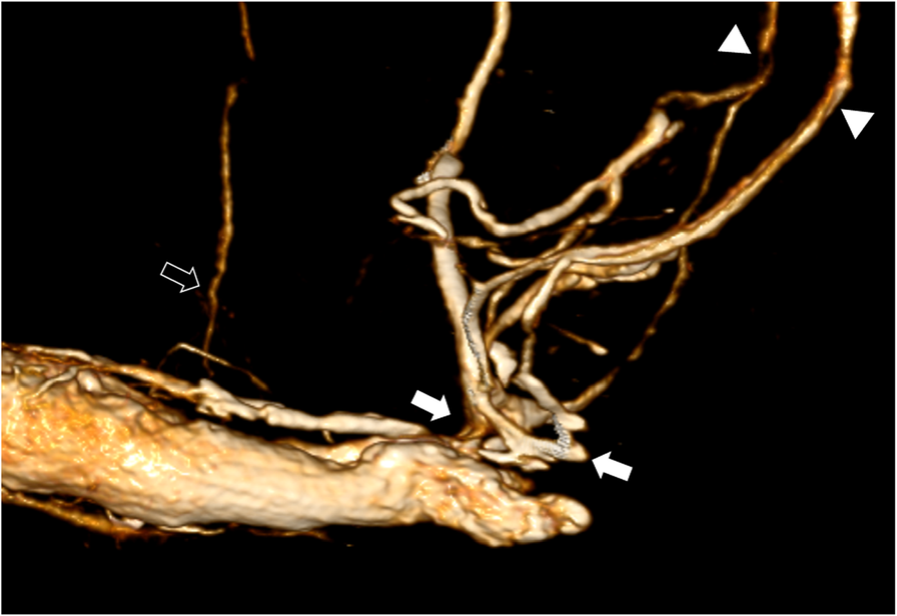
Contrast enhanced computed tomography cavernosography (volume rendering) demonstrating major venous leakage from deep dorsal penile veins via periprostatic veins (arrows) draining into internal pudendal veins and bilateral iliohypogastric veins (arrowheads). Besides there is minor venous leakage originating more peripherally via superficial inferior epigastric veins (open arrow)
